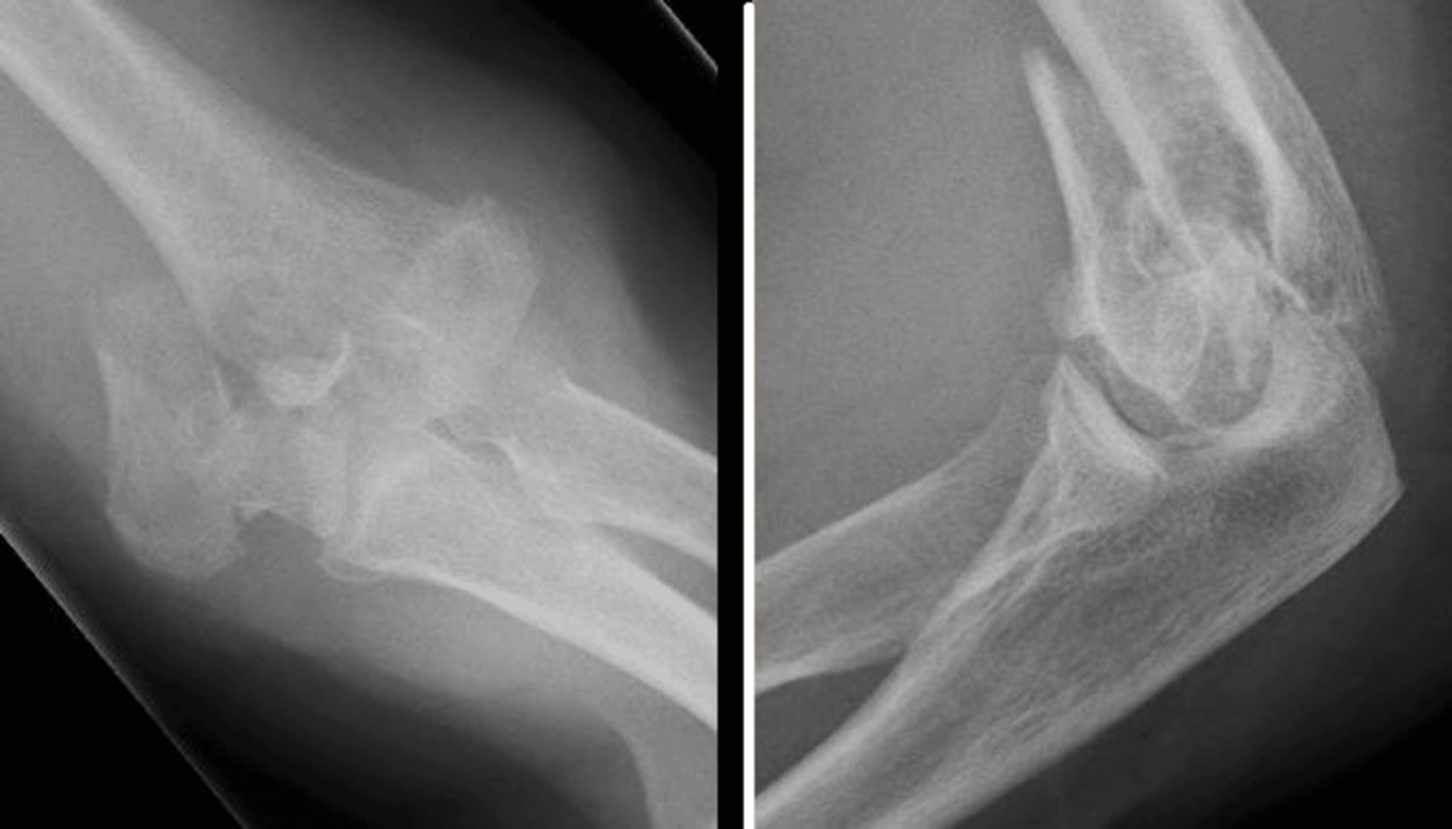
Preoperative radiograph of a patient's elbow who underwent TER later. TER: total elbow arthroplasty
